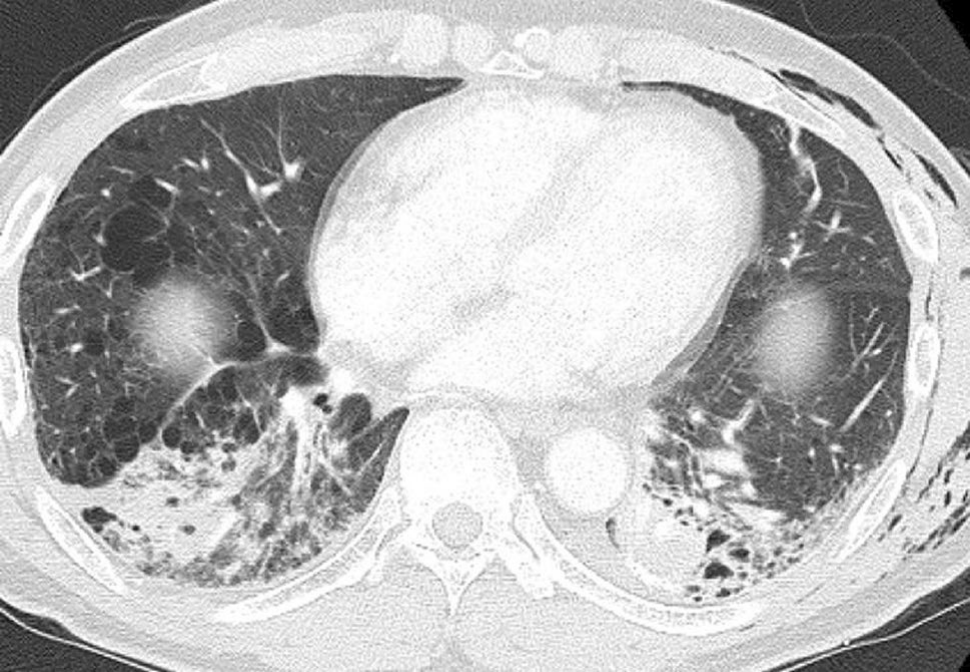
Pneumonia following left lower lobe wedge resection. The patient developed oxygen desaturation on postoperative day 1. Initial CXR was suggestive of pneumonia, and same-day CT imaging confirmed the diagnosis of aspiration pneumonia. CT revealed bilateral consolidation and ground-glass opacities predominantly in the lower lobes, consistent with aspiration pneumonia
